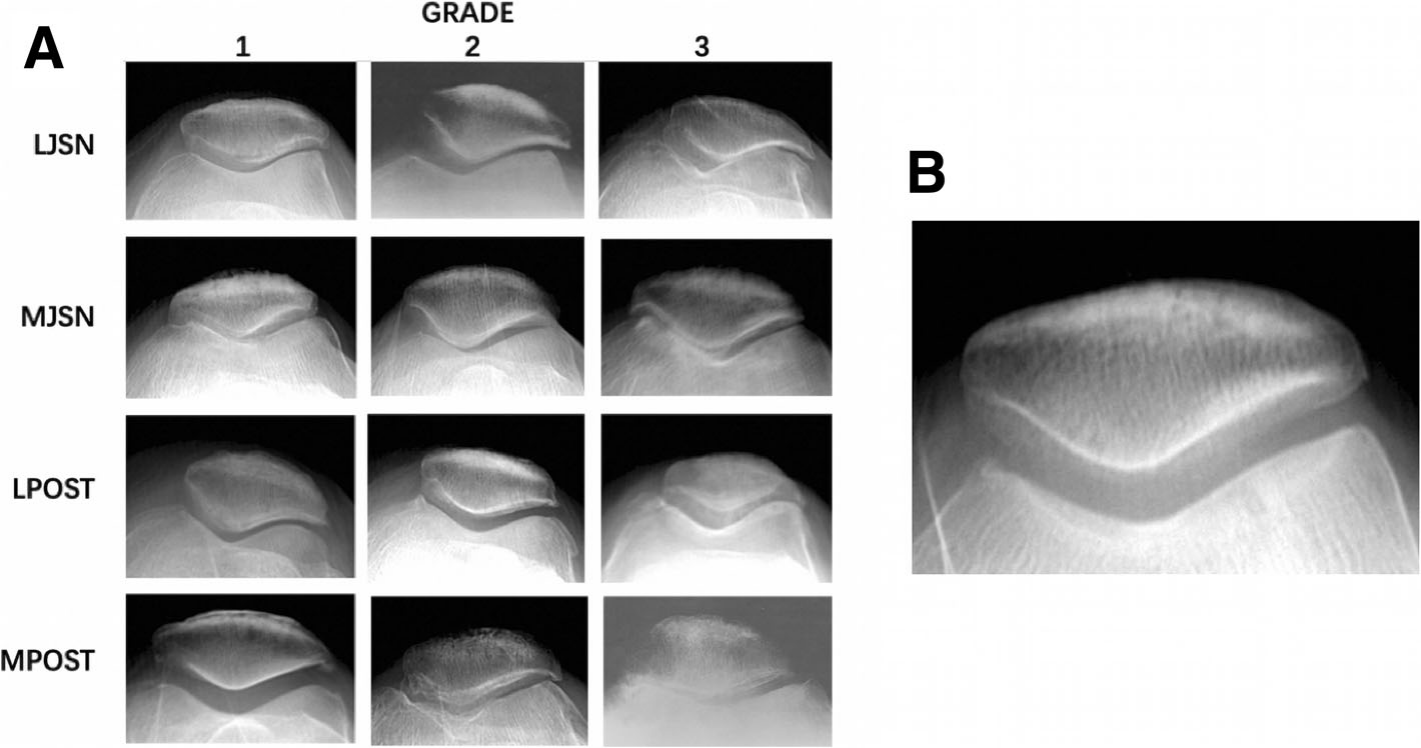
The normal radiograph (b, grade 0) is contrasted to grade1, grade2, and grade 3 abnormal radiographic features (a) on the skyline view of the patellofemoral joint Abbreviations. LJSN: lateral joint space narrowing; MJSN: medial joint space narrowing; LPOST: lateral patellofemoral osteophyte; MPOST: medial patellofemoral osteophyte.
